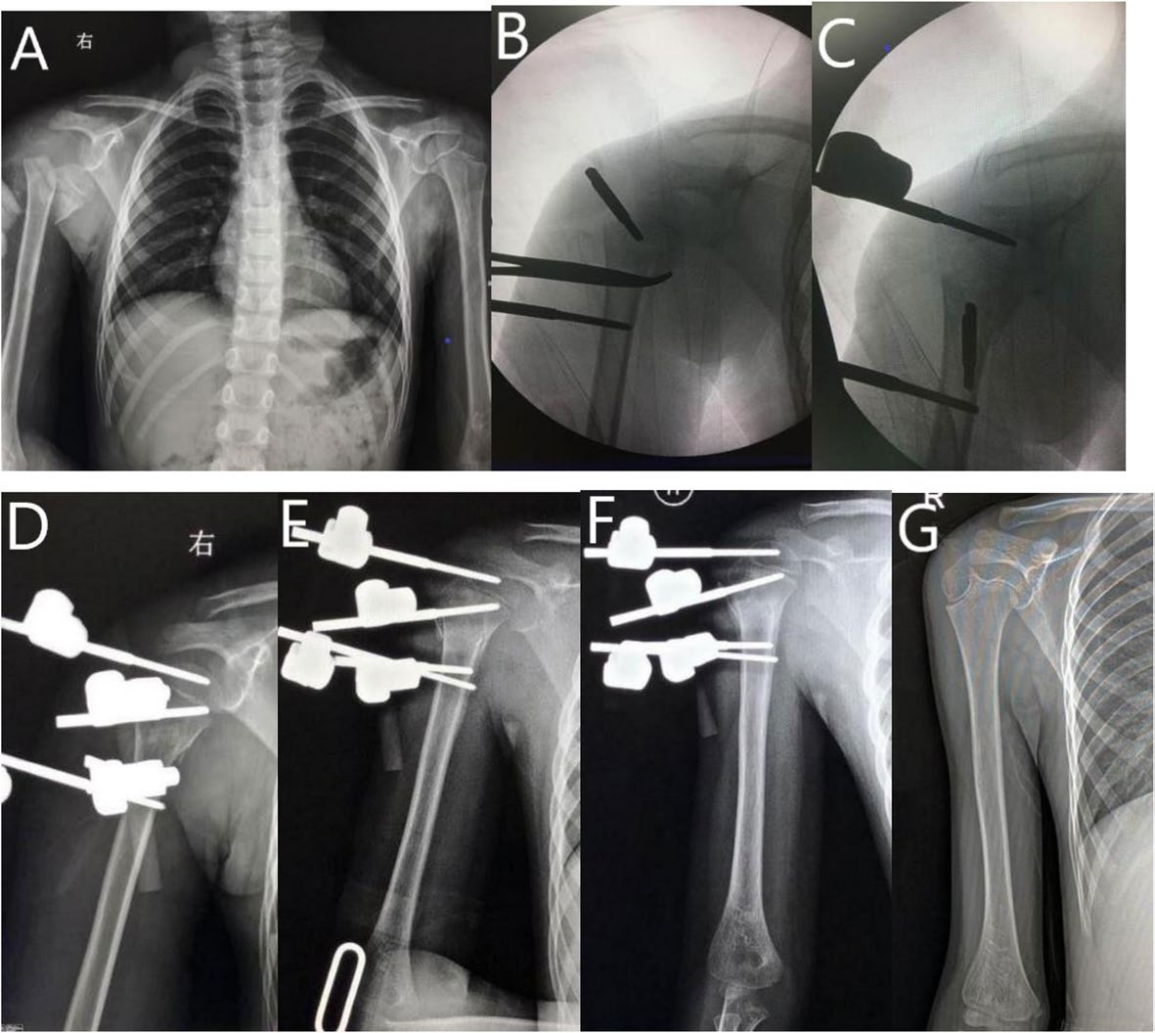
6-year-old girl with proximal humeral epiphyseal SH II fracture and shoulder dislocation. A Radiograph of humerus before surgery. B Radiograph of intraoperative reduction. C Radiograph of the shoulder joint after reduction. D Radiograph of the shoulder joint after surgery. E Radiograph of the humerus at 1-month follow-up. F Radiograph of the humerus at 2-month follow-up. G Radiograph of the humerus at 4-year follow-up
